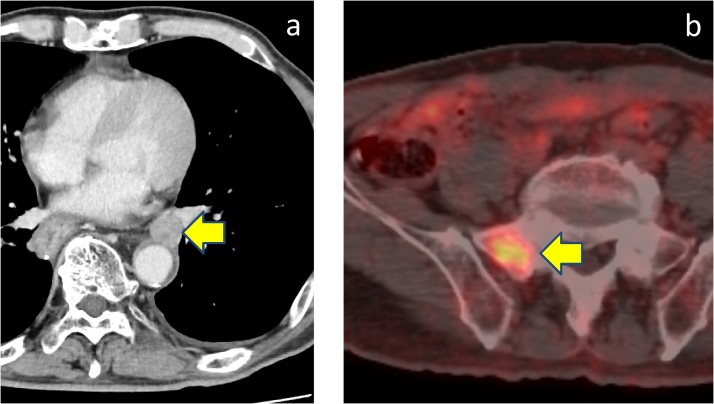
PET-CT showed new metastasis in hilar lymph node and sacrum.
